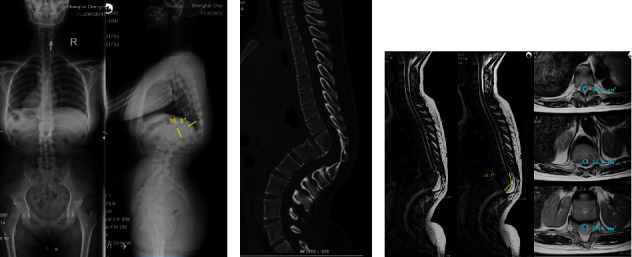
A 45-year-old female with spinal tuberculosis presented with 96.8° of kyphosis (a, AIS grade E). (b) CT revealed fusion of T11 and T12 vertebrae. (c) T2-weighted MRI showed tortuous spinal cord in the kyphotic region. SDR was 23.4%. SCA was 127.7°. CSAR was 13.4%. SDR: spinal cord sagittal diameter ratio; SCA: spinal cord angle; CSAR: spinal cord cross-sectional area ratio.
